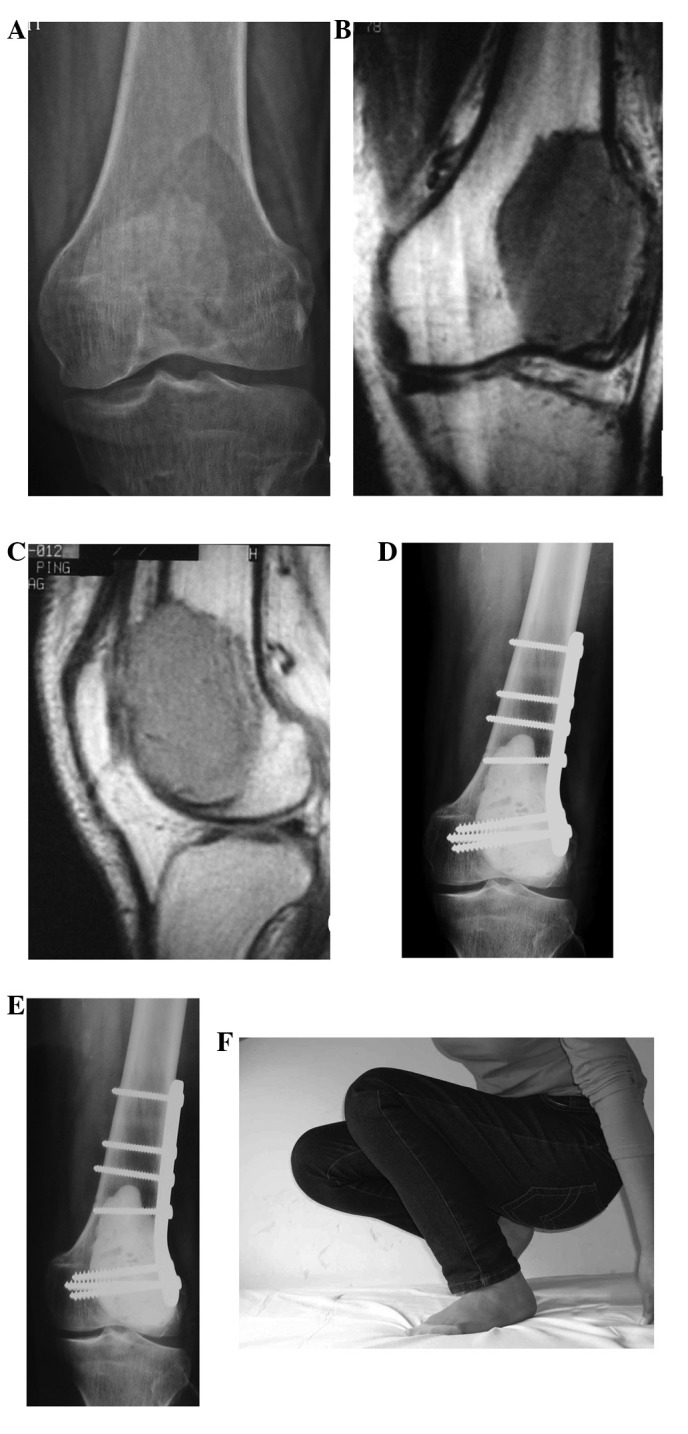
A 30-year-old female patient with giant cell tumor (GCT) of the left distal femur. (A) X-ray film reveals osteolytic destruction and cortical thinning at the left femoral condyle. (B) and (C) T1-weighted MRI reveals low signal at the distal femur and a lateral visible tumor penetrating the front side of the cortex. (D) 4 days after surgery, postoperative X-ray reveals bone cement filling and internal fixation are normal. (E) and (F) 22 months after surgery, X-ray shows that the joint space is normal, no lucent zones surround the bone cement, internal fixations are firm and the joint function is normal.
